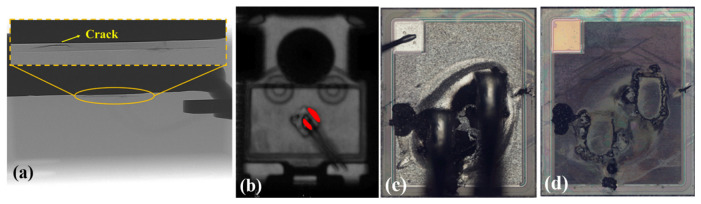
DUT-AT3: (a) X-ray inspection result. (b) SAM inspection result. (c) Surface morphology after decapsulation and before Al removal. (d) Surface morphology after decapsulation and Al removal.
